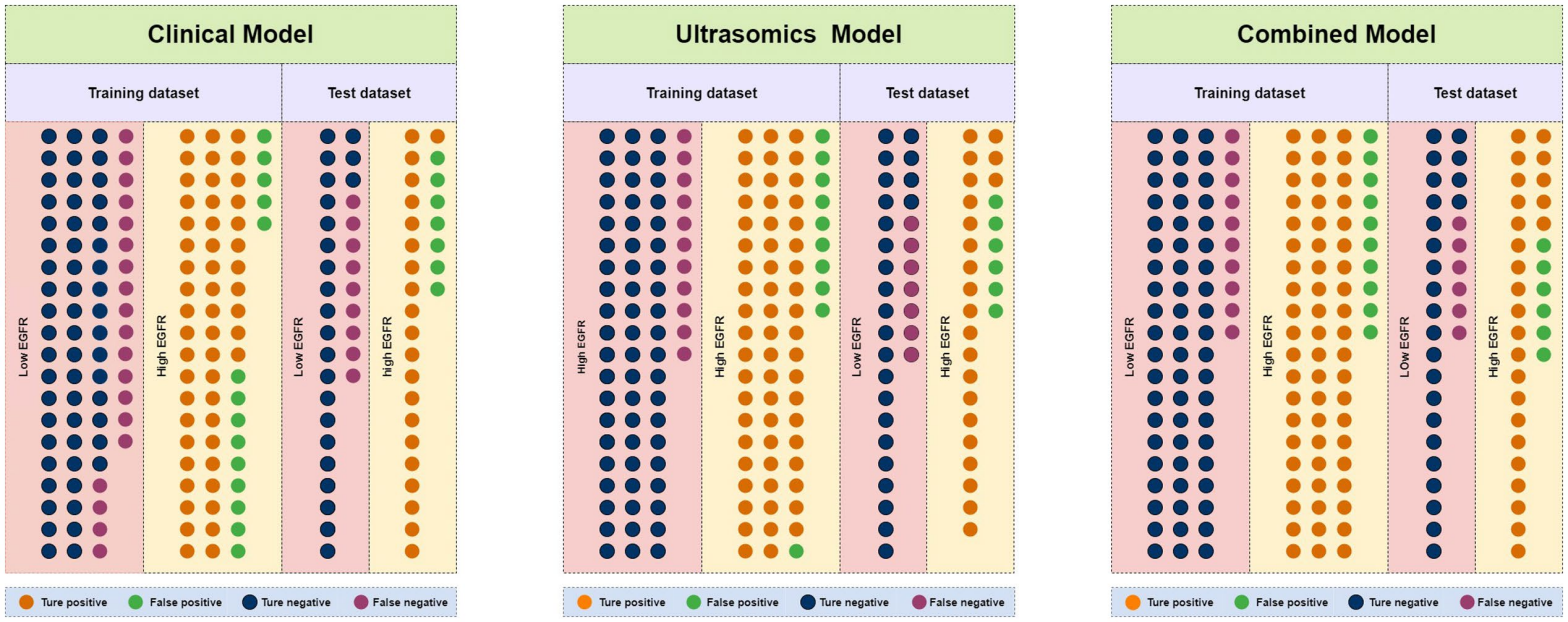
Number of true positive, false positive, true negative, and false negative events in the training and test dataset for clinical models, ultrasomics models, and combined models of RF algorithm.
